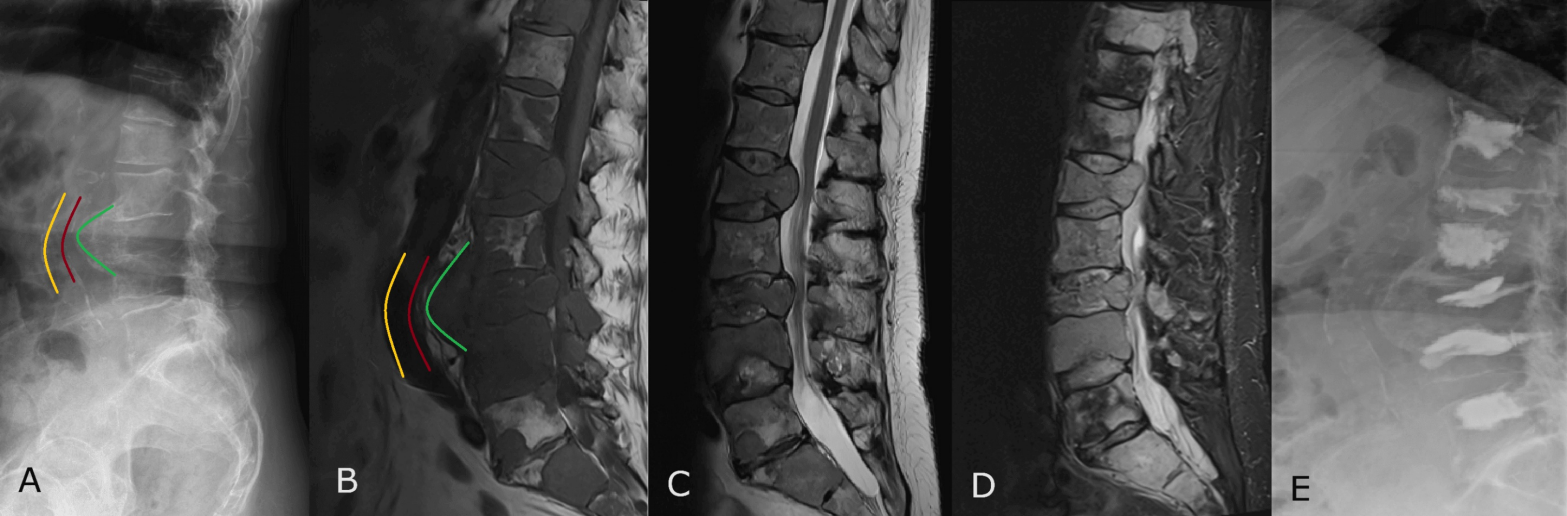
Pre- and postoperative images of multiple spinal metastases A) Preoperative radiographic evaluation showed an osteolytic lesion in the L3 vertebral body that compressed the large vessels anterior to it (the yellow line outlines the anterior wall of the large vessel, the red line outlines the posterior wall of the large vessel, and the green line outlines the anterior margin of the osteolytic lesion); B) T1-weighted MRI sagittal sequence correlated with the X-ray image (see the legend above); C) T2-weighted MRI sagittal sequence demonstrated the precise contours of the vertebral bodies; D) short tau inversion recovery (STIR) MRI sagittal sequence showing multiple vertebral metastases; E) Radiographic evaluation at postoperative day seven showed the distribution of bone cement with no extrusion towards the spinal canal
